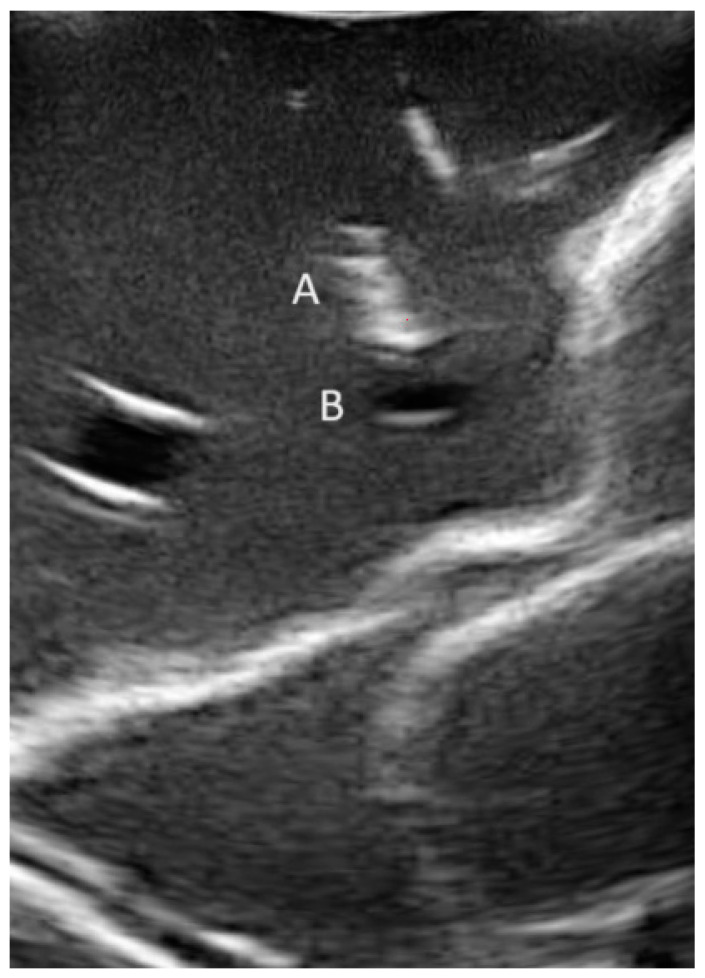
Conventional sonography-guided puncture in out-of-plane technique. A: needle; B: common femoral artery (CFA).
